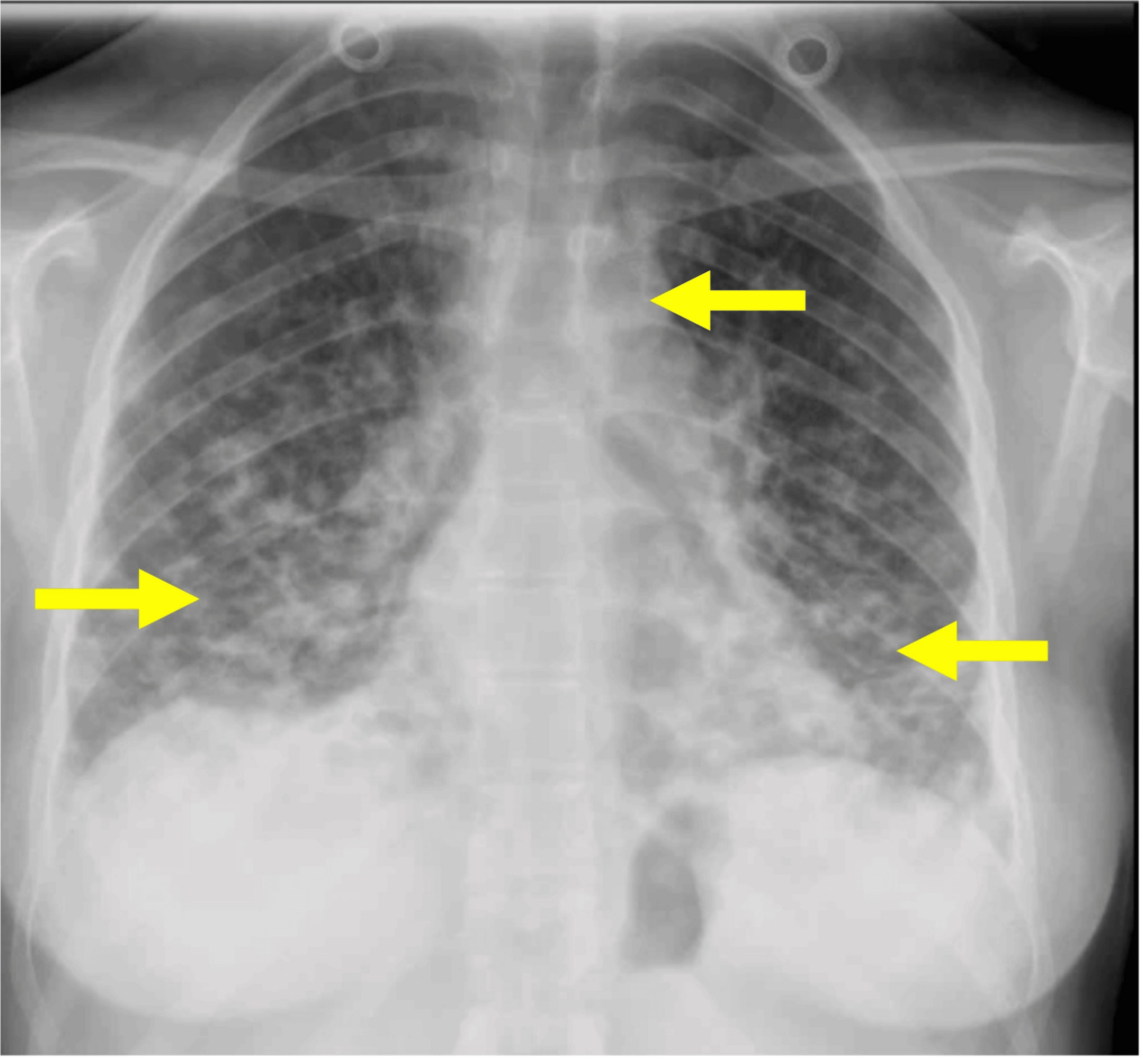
Posteroanterior chest X-ray Bibasal infiltrates and mediastinal enlargement (arrows)
